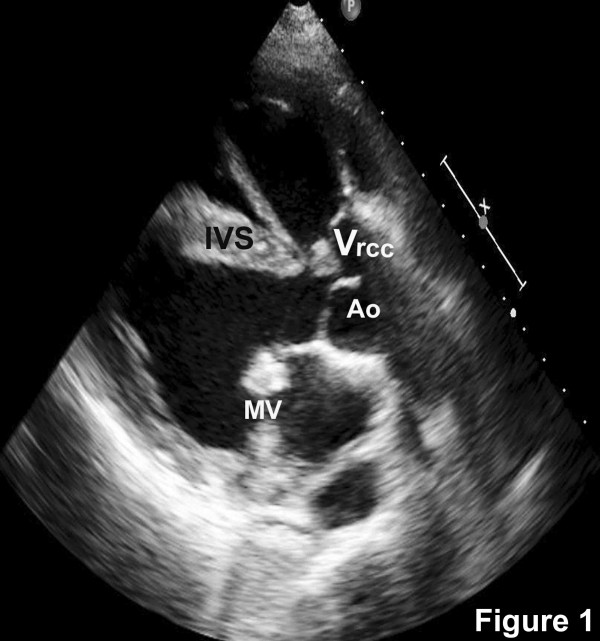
Parasternal long axis view showing the malaligned ventricular septal defect, aortic override (Ao), vegetation on the right coronary cusp (V_rcc_) and thickened mitral valve (M).
